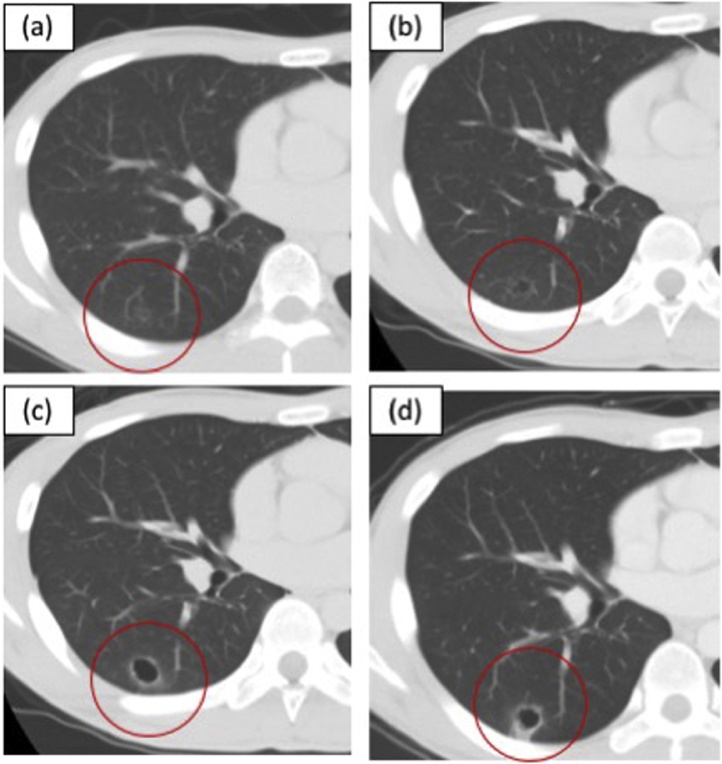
a CT showing a ground glass opacity-lesion in the right S6 segment. b A thin-walled cavity appeared in the center of the lesion. c The cavity extended, and its wall thickened. d The GGO-like lesion with the cavity gradually increased in size.
